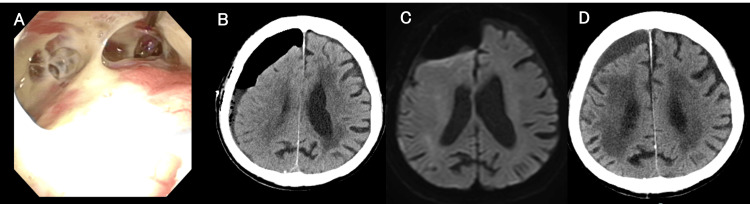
Intraoperative findings (A) and postoperative imaging findings (B-D) of endoscopic abscess removal A: A purulent collection was observed in the subdural space. B: Head CT confirmed that the subdural abscess had been largely evacuated. C: Brain MRI-DWI confirmed that the abscess had been substantially removed. D: Head CT on day 48 reveals no evidence of subdural abscess recurrence. CT, computed tomography; MRI, magnetic resonance imaging; DWI, diffusion-weighted imaging
